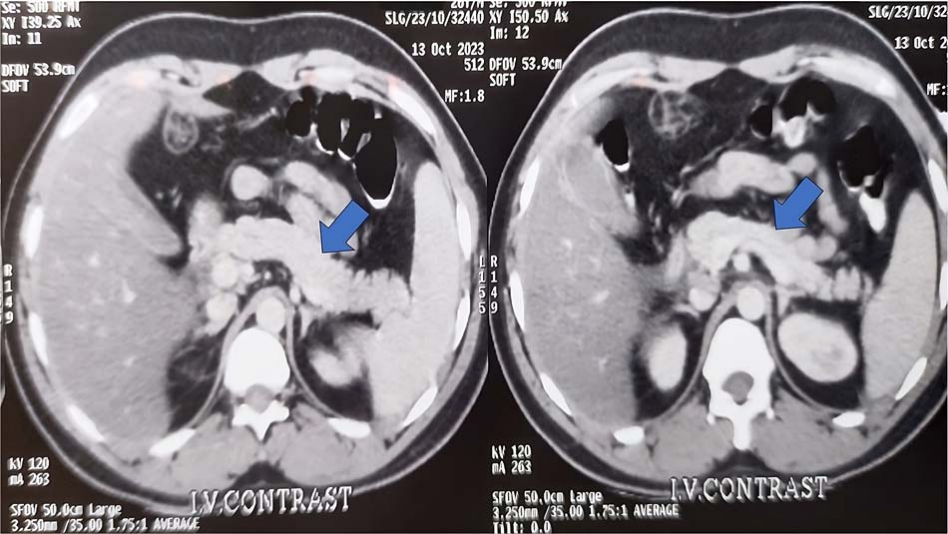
Contrast-enhanced computed tomography of the abdomen shows hepatosplenomegaly and a bulky pancreas with peripancreatic fat stranding and collection with thickening at the right anterior conal fascia and lateral conal fascia.
